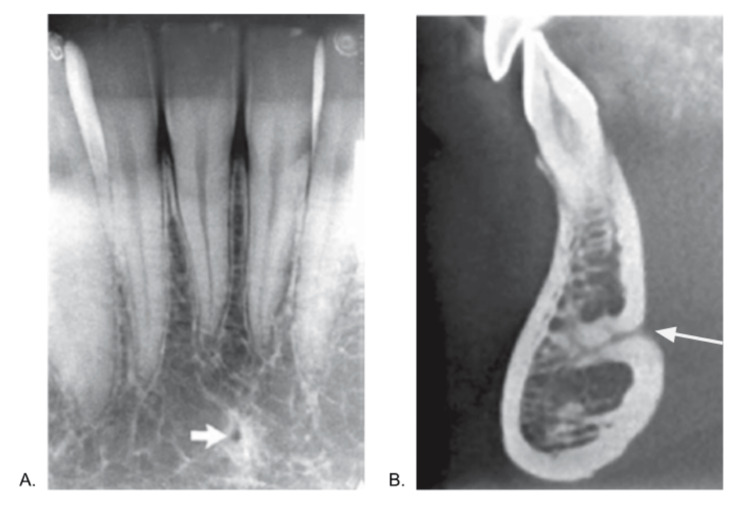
a: The lingual foramen, indicated by the arrow, is the small rounded opening shown in the intraoral periapical view. b: In the cross-sectional cone beam computed tomography image, the lingual foramen extends deep into the mandible from the lingual surface. Reproduced from reference [10] under the Creative Commons License (CC BY-NC-SA 4.0). Labels (A and B) were adjusted from the original labels to align with the current study, and an arrow was added to panel B. No other modifications were made to the image. For further details, please visit the Creative Commons License (https://creativecommons.org/licenses/by-nc-sa/4.0/).
